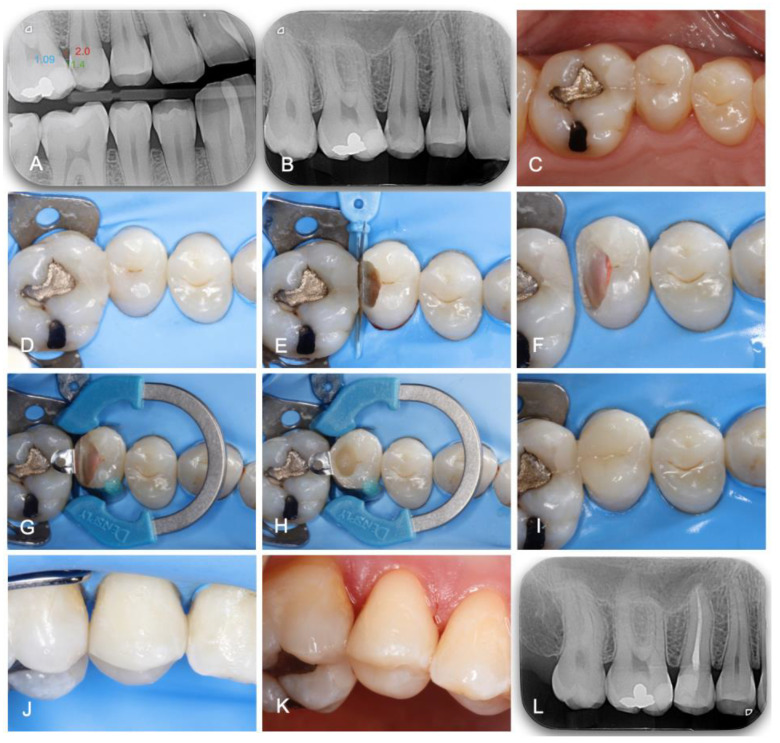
Clinical case where both endodontic and restorative procedures were performed in one appointment. (A) Bite-wing radiography and embrasure measurement. (B) Preoperative periapical radiography. (C) Preoperative view. (D) Isolation. (E) Pre-wedging and adjacent tooth protection. (F) Root canal treatment. (G) Placement and stabilization of the matrix. (H) Class II transformation into Class I. (I–K) Final view. (L) Final bite-wing radiography.
